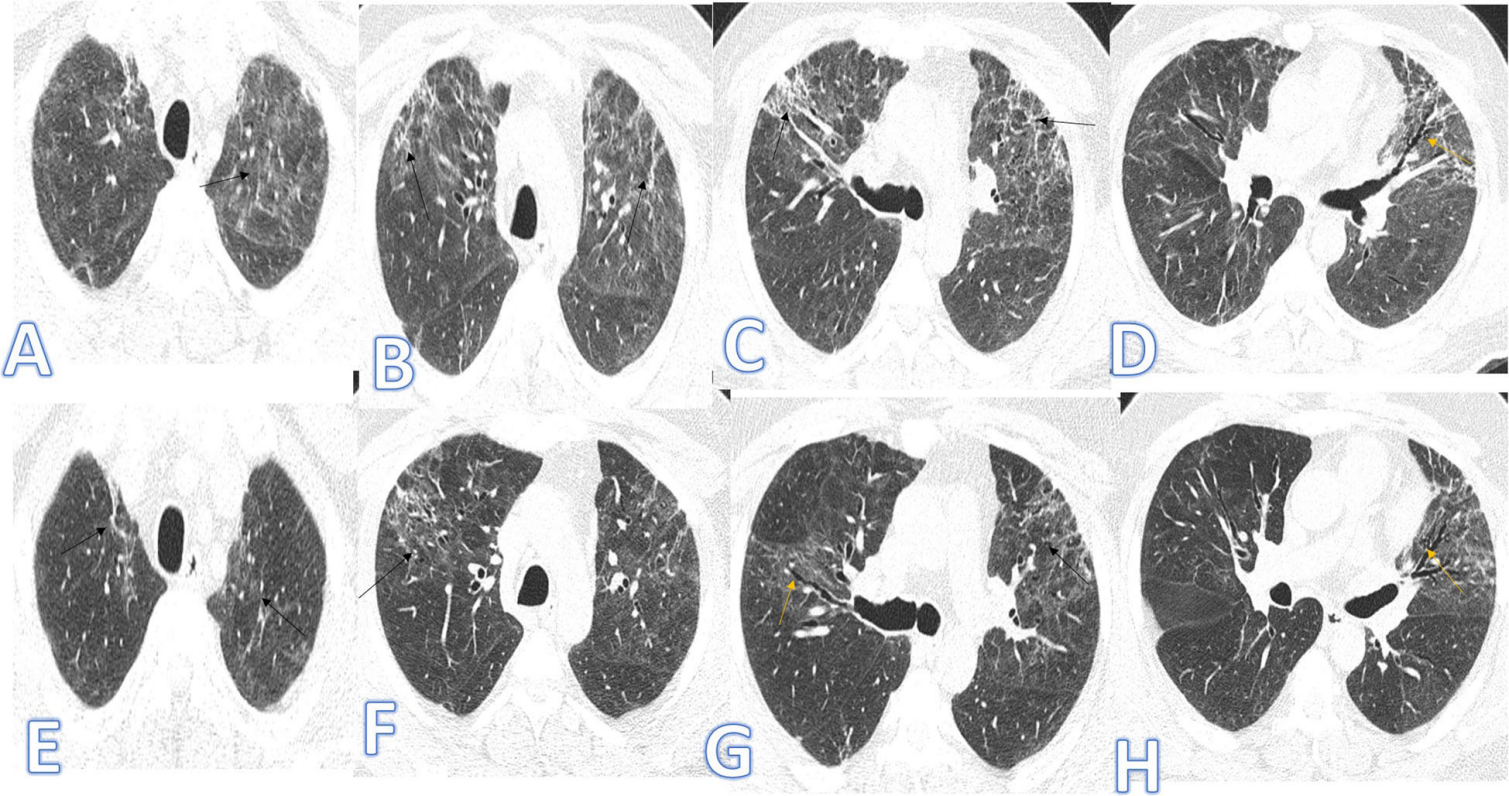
Forty-three-year-old male, he has no past medical illness; peak CT severity score 18; consolidation/crazy-paving score 14. He was admitted to the ICU; laboratory results showed lymphopenia, high CRP, d-dimer, and serum ferritin. Steroid was given; length of stay during hospitalization is 65 days. CT after 3 weeks of admission (a–d) showed bilateral GGO with bilateral upper lobe fibrotic bands (arrows) and traction bronchiectasis (orange arrows). Follow-up CT 2 months after discharge (e–h) showed lung fibrosis with parenchymal bands, coarse reticular pattern (arrows), and mild traction bronchiectasis at the anterior segments of both upper lobes (orange arrows).
